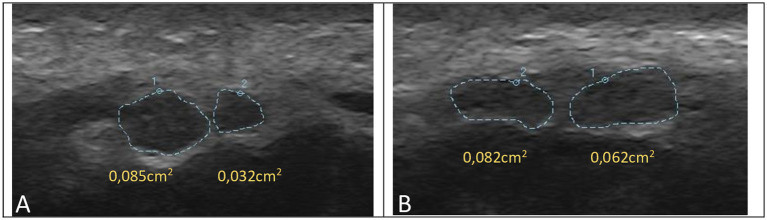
Ultrasonographic examples of bifid median nerve. (A) Median nerve cross-sectional area in a patient with mild CTS. (B) Median nerve cross-sectional area in a patient with minimal CTS.
